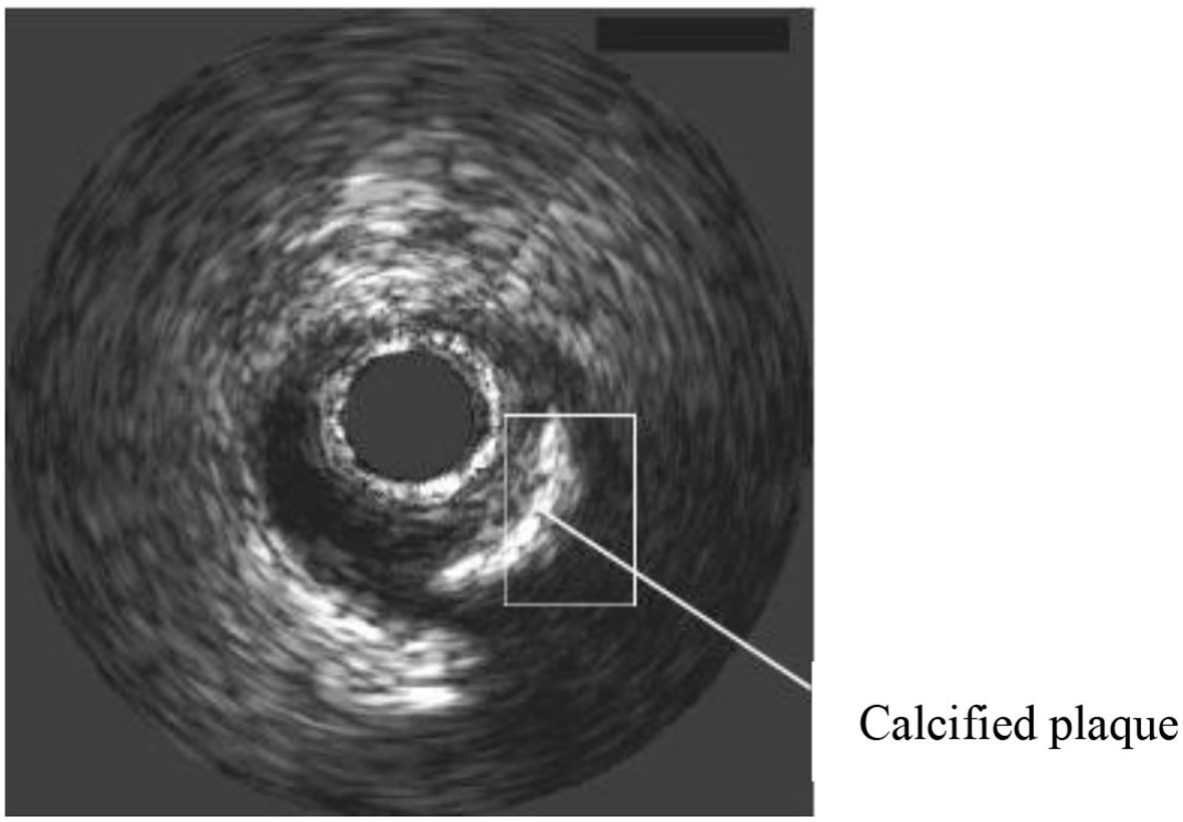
Ultrasound vascular acoustic shadow.
